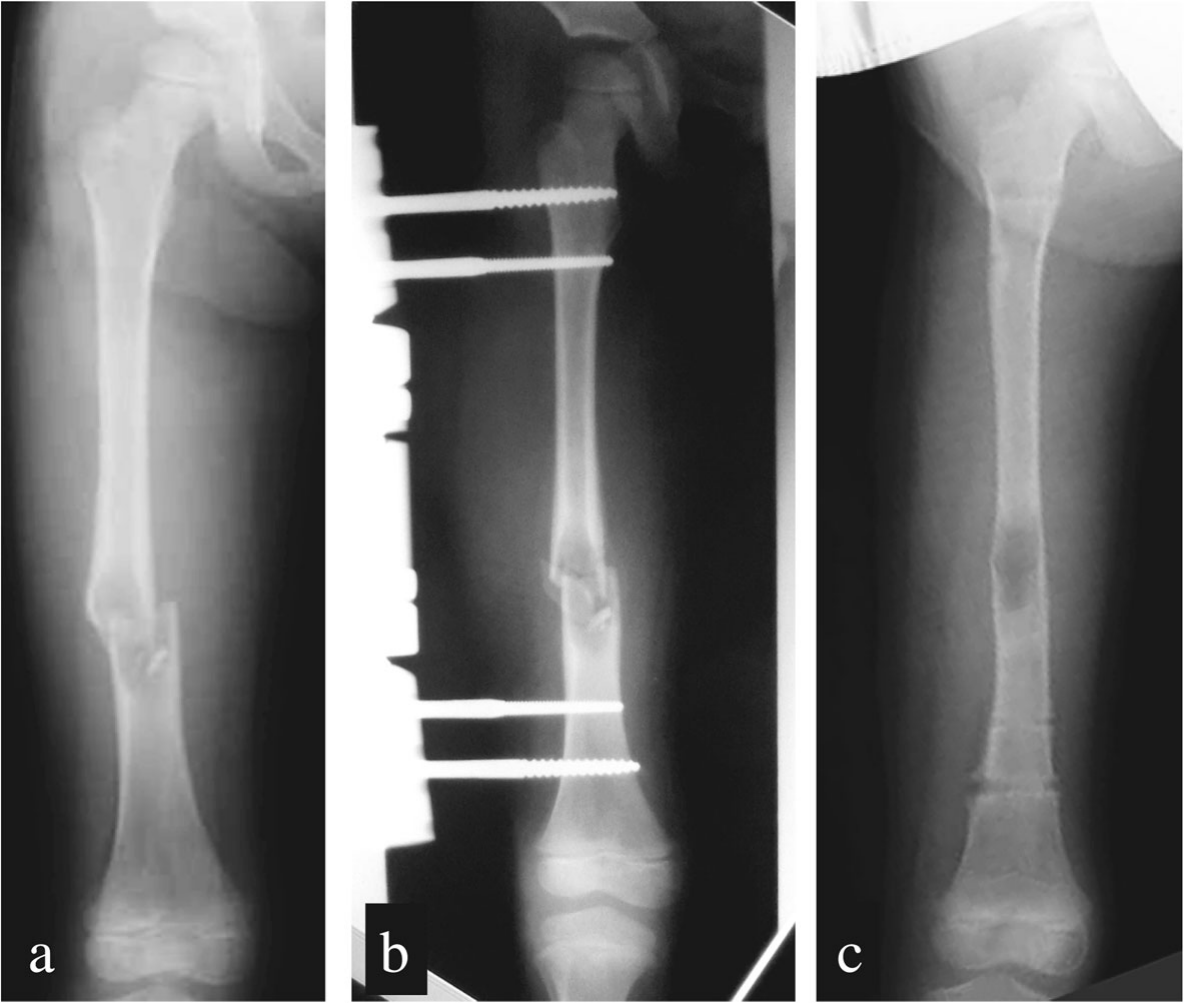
Right femur frontal radiographs. a Pathological bone fracture in the right femur at age 4 years, 6 months. b After lesion curettage/external fixation; (c) bone fusion and removal of the fixator
